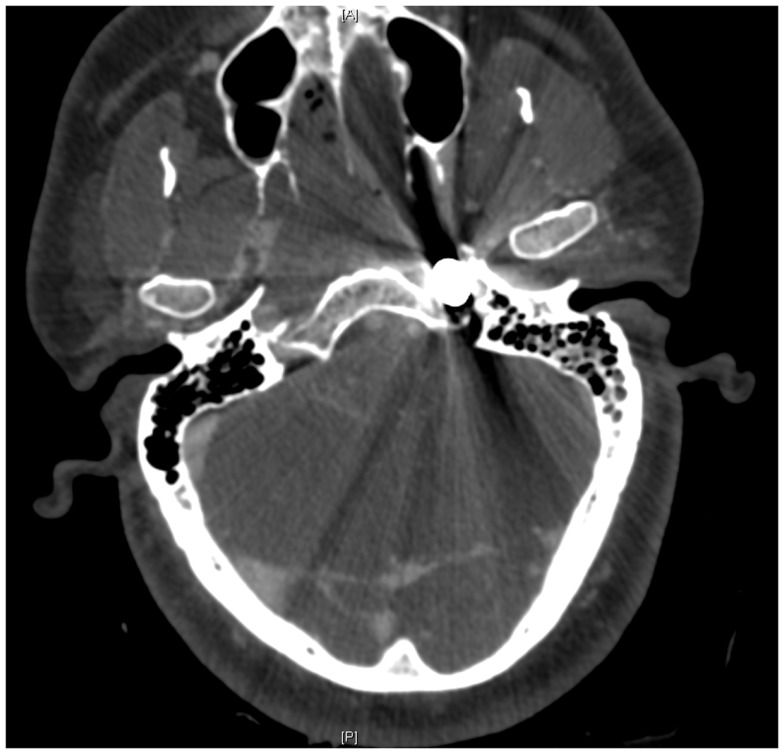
Pre-operative CT scan showing complete transection of the petrous portion of the left internal carotid artery.
